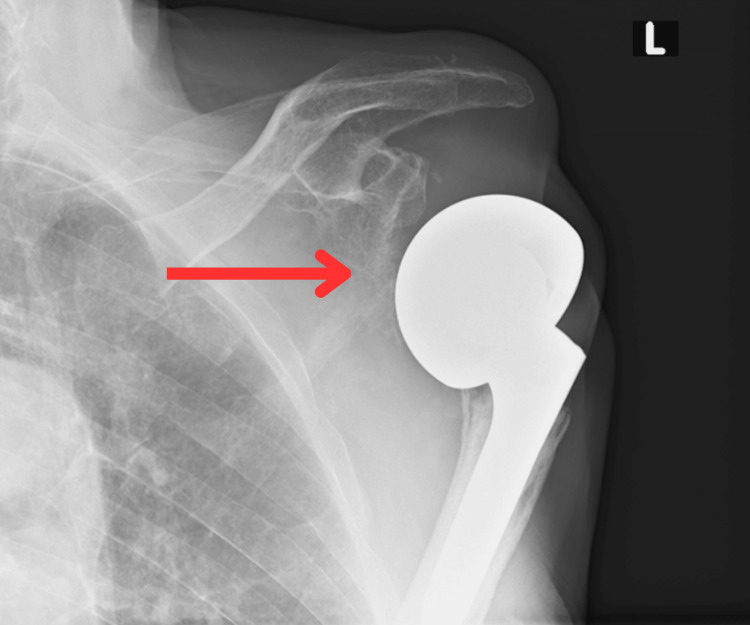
Post-operative radiograph following conversion to large-head bipolar hemiarthroplasty
